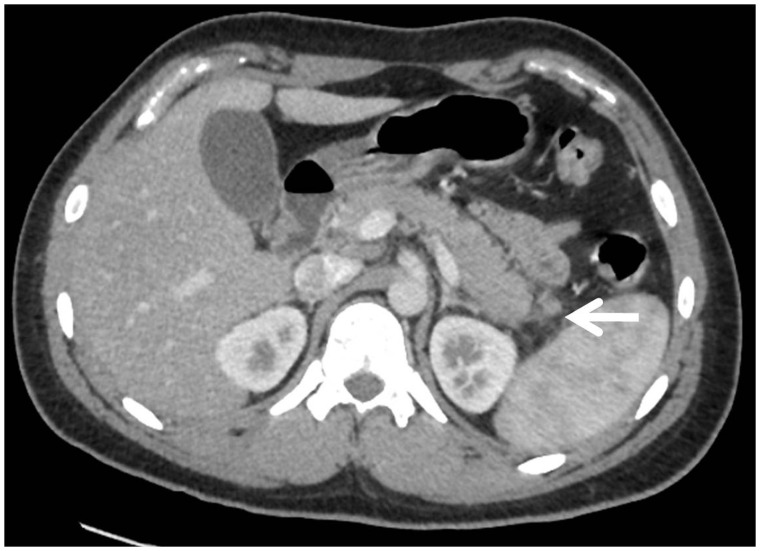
Axial contrast-enhanced CT scan in a 22-year-old male patient with interstitial oedematous pancreatitis, showing mildly increased bulk in pancreatic tail region with peripancreatic fat stranding (arrow).
